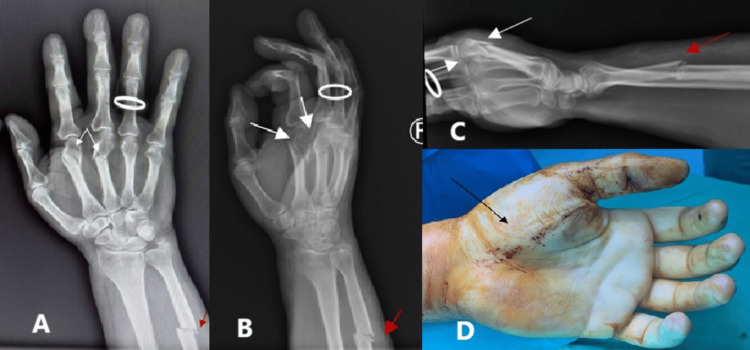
Preoperative (A) anteroposterior, (B) oblique, and (C) lateral X-ray views show fractures of the second and third metacarpals (white arrows) and a fracture of the distal ulna (red arrow). (D) The palmar surface shows significant swelling in the thenar region of the right hand (black arrow).
